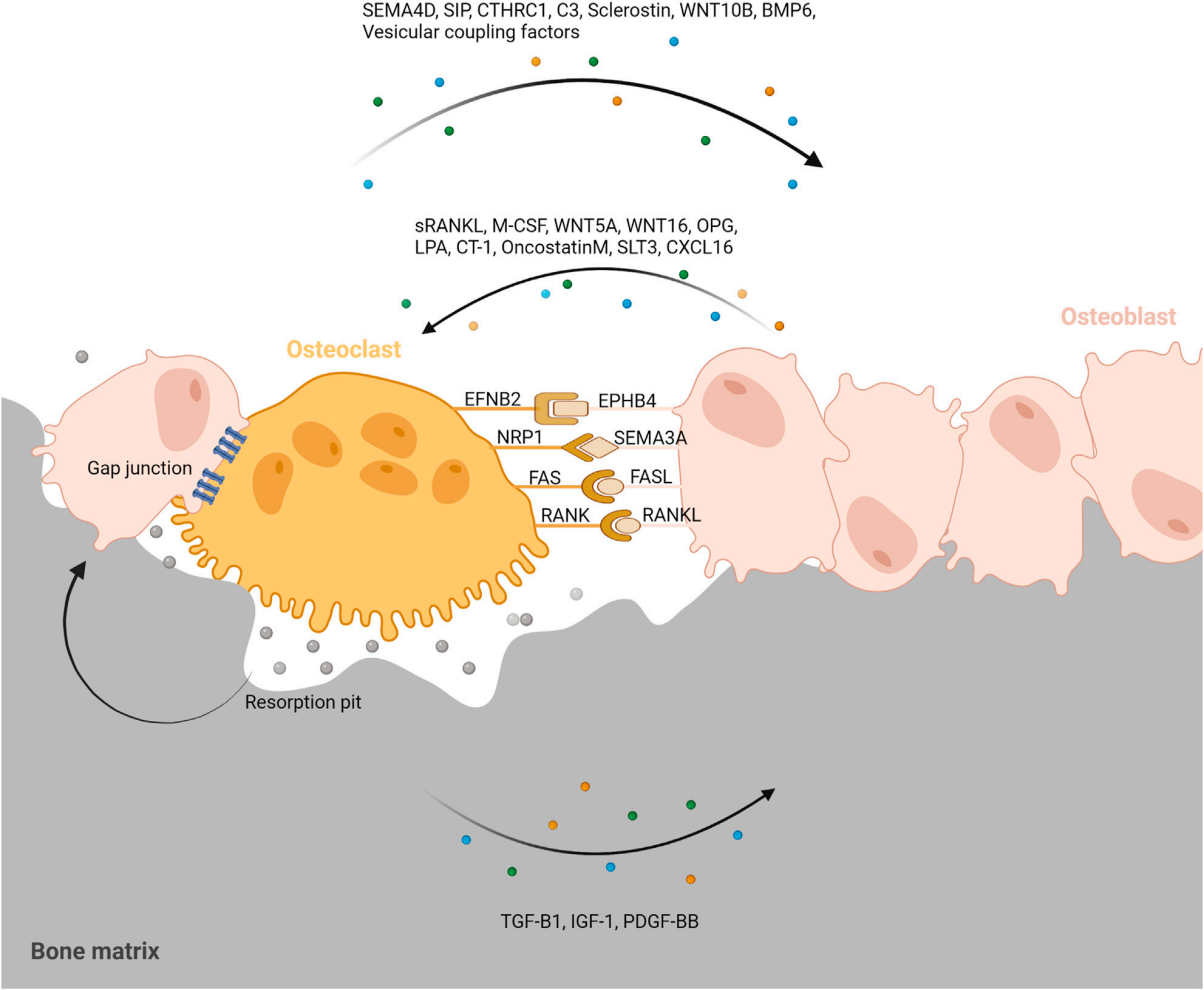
Coupling signals between osteoclast and osteoblast. To accomplish coordination between bone formation and resorption during bone remodeling, communication between osteoclasts and osteoblast lineage cells, as well as interactions with the resorption pit and others are essential. Coupling signals between osteoclast and osteoblast can be classified into five categories; The membrane-bound coupling signals are mainly composed of five coupling signals: gap junction, EFNB2/EPHB4, NRP1/SEMA3A, FAS/FASL, RANK/RANKL; Soluble coupling signals released from osteoblast mainly refer to the soluble factors acting on osteoclasts released by osteoblasts such as sRANKL, M-CSF, WNT5A, WNT16, OPG, LPA, CT-1, OncostatinM, SLT3, CXCL16; Soluble coupling signals released from osteoclast mainly refer to the soluble factors acting on osteoblasts released by osteoclasts such as SEMA4D, SIP, CTHRC1, C3, Sclerostin, WNT10B, BMP6, Vesicular coupling factors; Matrix-derived coupling signals are factors released by matrix which is mediated by osteoclast degradation during bone resorption such as TGF-B1, IGF-1, PDGF-BB; Vesicular coupling signals mainly refers to exosomes, microvesicles, and apoptotic bodies. These membrane-containing EVs can deliver proteins, lipids and mRNAs through exocytosis and endocytosis to promote coupling. Furthermore, the resorption pit, cellular canopy, and reversal phase could also play a role in the coupling between osteoclast and osteoblast.
